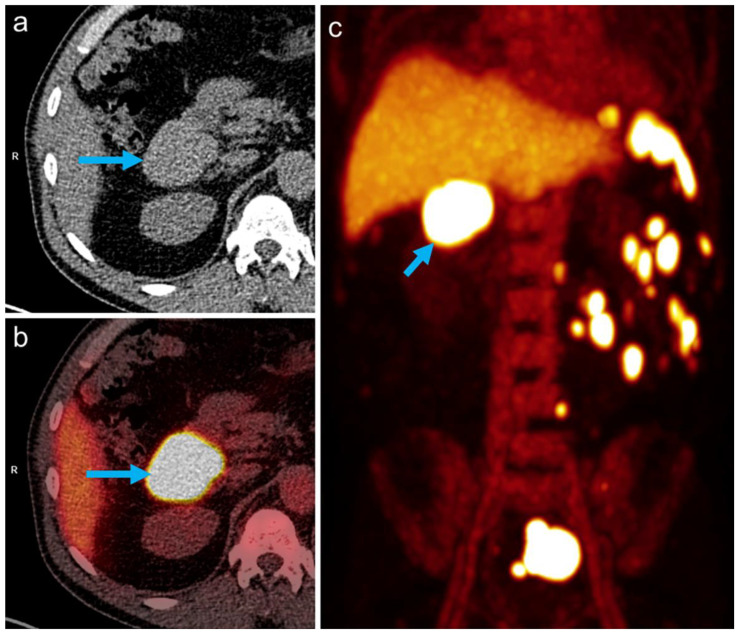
Multiple masses with high tracer uptake seen on RBC-PET/CT (a): non-contrast CT, (b): PET/CT fusion, (c): PET maximum intensity projection (MIP) image acquired 25 min after tracer injection).
